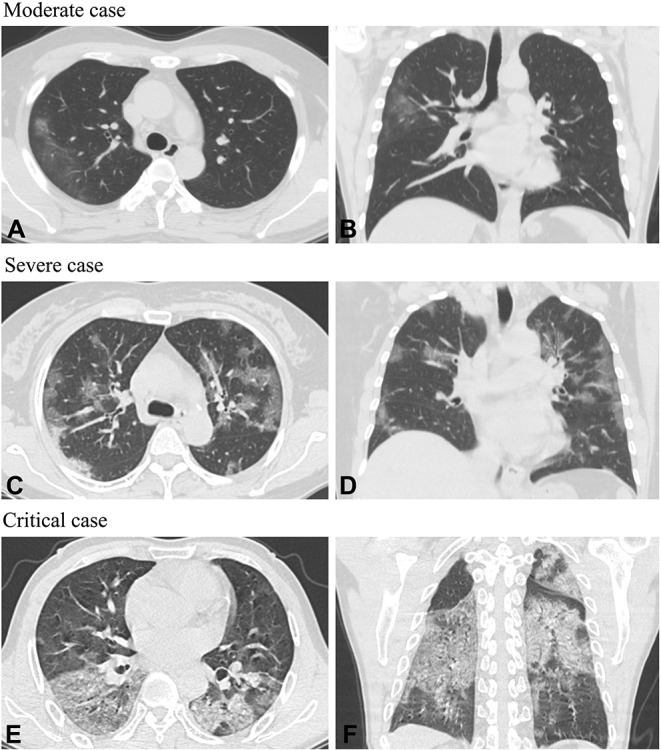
Axial planes and coronal chest CT scans in patients with COVID-19. Moderate case: (A,B) Chest CT images of a 41-year-old man showed a ground-glass lesion in the right lobe on the 3rd day following a fever. Severe case: (C,D) Chest CT images of a 55-year-old woman showed bilateral multifocal ground-glass opacities on the 8th day after having chills, cough, and expectoration. Critical case: (E,F) Chest CT images of a 61-year-old man showed diffuse patchy shadowing and mixed consolidation on the 13th day after having cough, expectoration, and fever.
